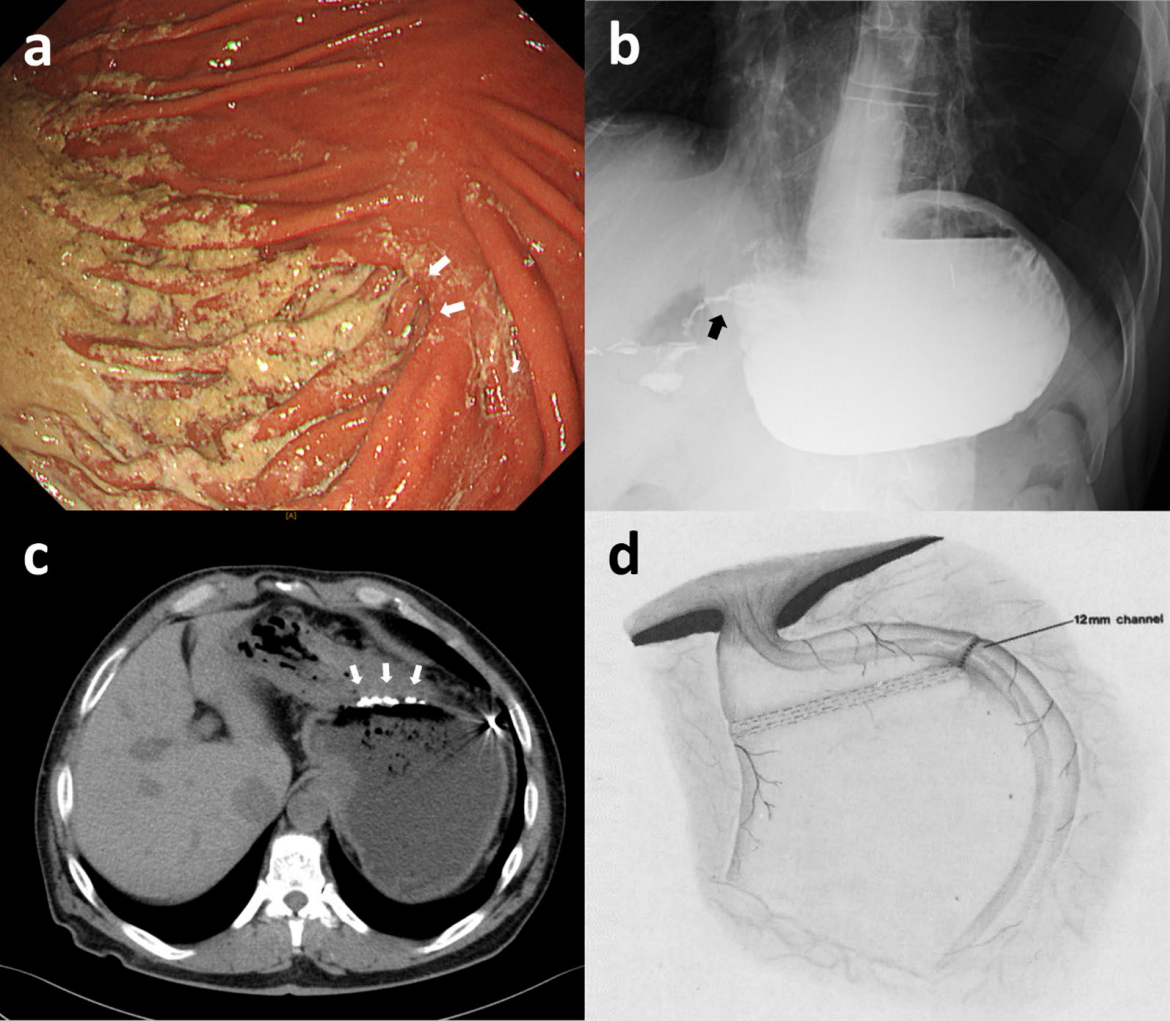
Preoperative findings. a Esophagogastroduodenoscopy showed a stoma (white arrows) on the greater curvature of the upper gastric body. b Fluoroscopy demonstrated retention of contrast medium in the fundus and poor outflow through the stoma (black arrow). c Abdominal computed tomography revealed a staple line (white arrows) at the upper gastric body and an enlarged fundus filled with food residue. d Complete image of Gomez gastroplasty (reproduced from ref. [4] with permission from Elsevier)
